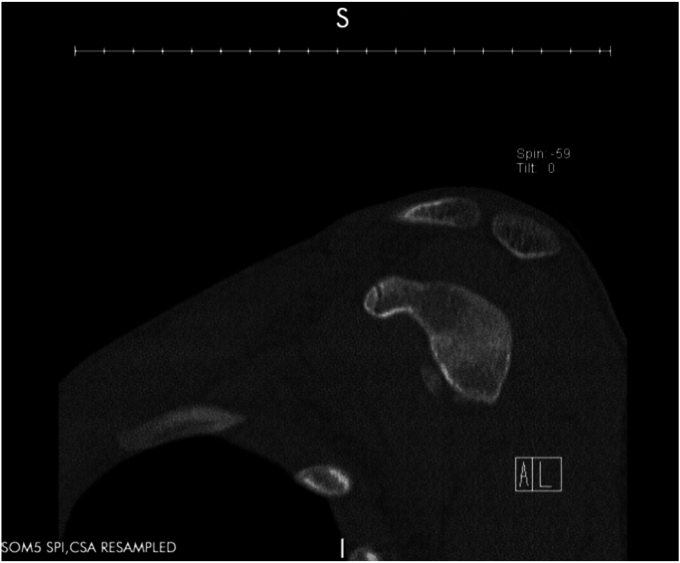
Sagittal CT image taken after anterior dislocation revealing bony Bankart lesion.
